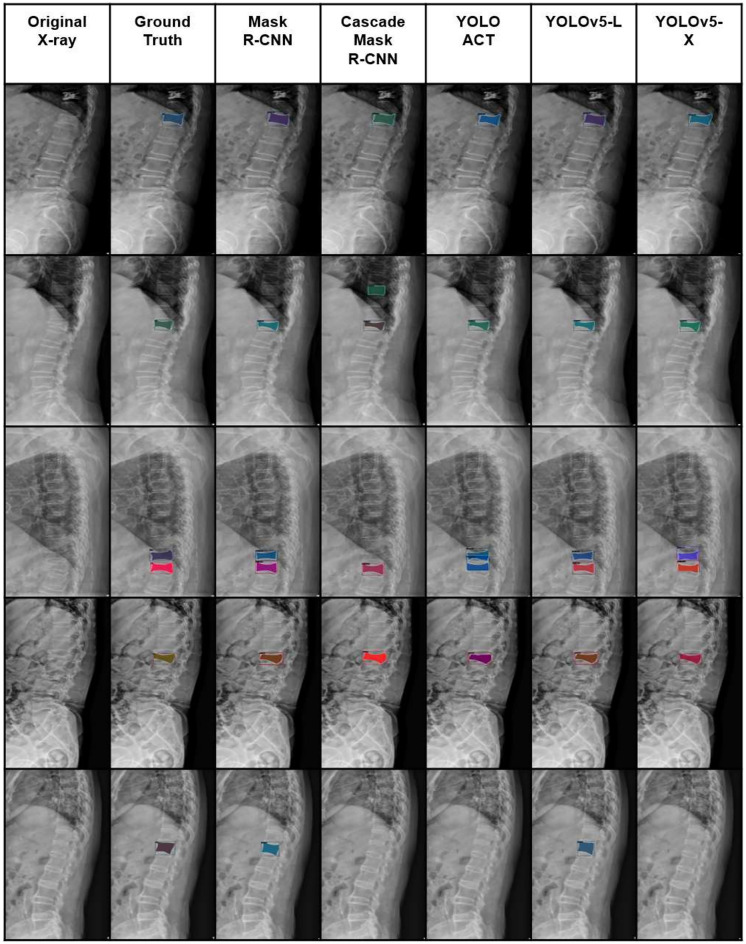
Examples of prediction results of each model. From left to right, actual spine lateral radiograph, ground-truth (expert-labeled fracture masks), and prediction from each model are shown.
